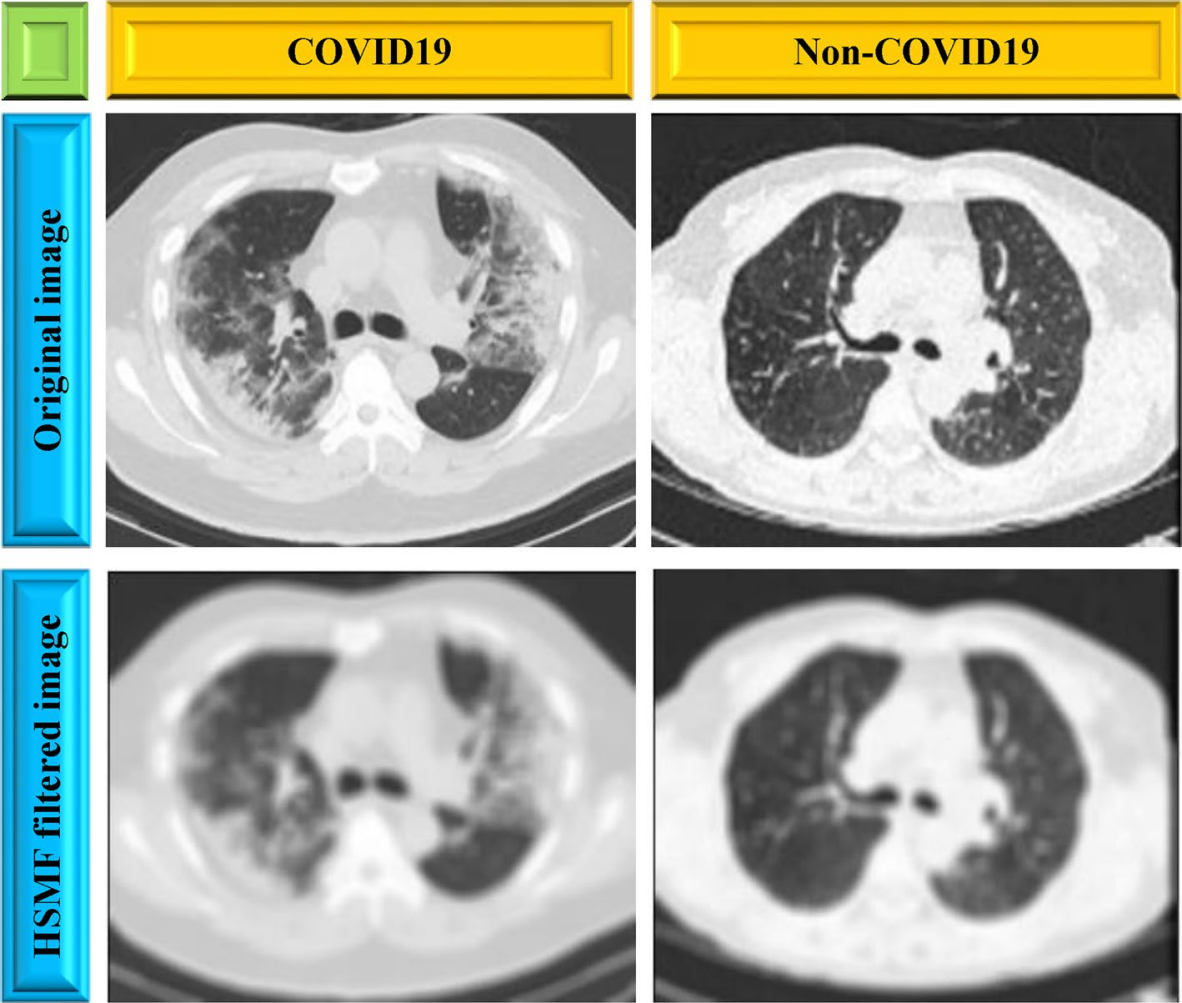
Filtered CT scan images using hybrid selective mean filter method.
